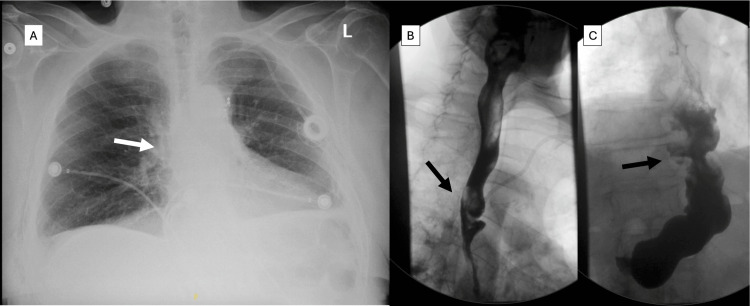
Imaging after esophagectomy Chest radiography showing no bulge in the right hilum (arrow, A). Esophagram shows a straight conduit at the anastomosis (arrow, B) and diaphragmatic hiatus (arrow, C)
